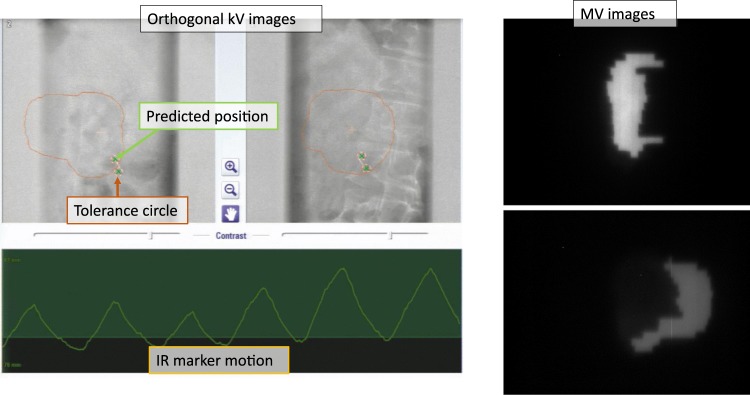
kV and MV X-ray monitoring images during DTT-IMRT. During DT-IMRT beam delivery, the predicted position of the internal fiducial marker (green x) and tolerance circle (a 3-mm radius) are superimposed on the orthogonal fluoroscopic kV images. The kV images are updated every 1 sec. When the predicted positions deviate from the actual fiducial marker positions, observers can consider the recalibration of the 4D model.
